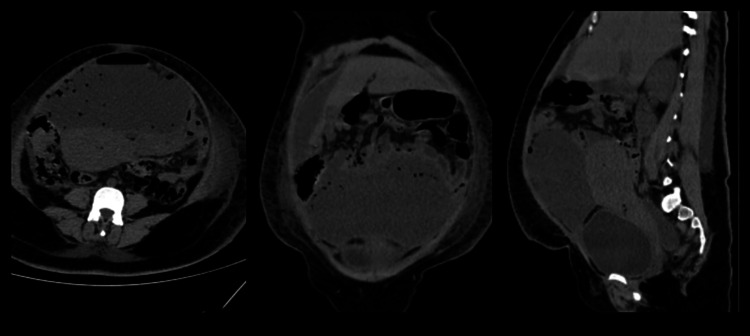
CT scan showing localized fluid collection anterior to the uterus, beneath the anterior abdominal wall, and superior to the bladder extending to the flanks with wall enhancement, highly suggestive of intra-abdominal abscess.
